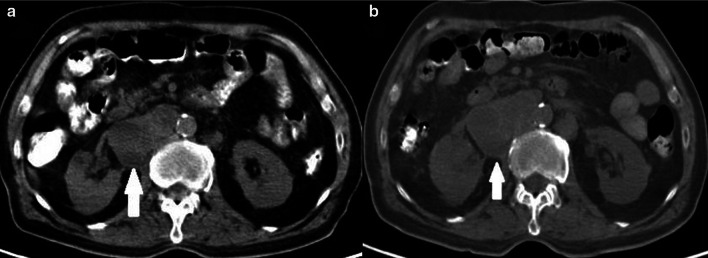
Stable disease, a 70-year old male patient with metastatic renal cell cancer under treatment with nivolumab (anti-PD-1 monoclonal antibody). a Axial CT image shows right paraaortic conglomerated mass like pathological lymph nodes measured 61.4 mm in diameter. b In the control CT examination performed even after one year, the size of the conglomerated mass like pathological lymph nodes were stable and measured 65.4 mm in diameter
